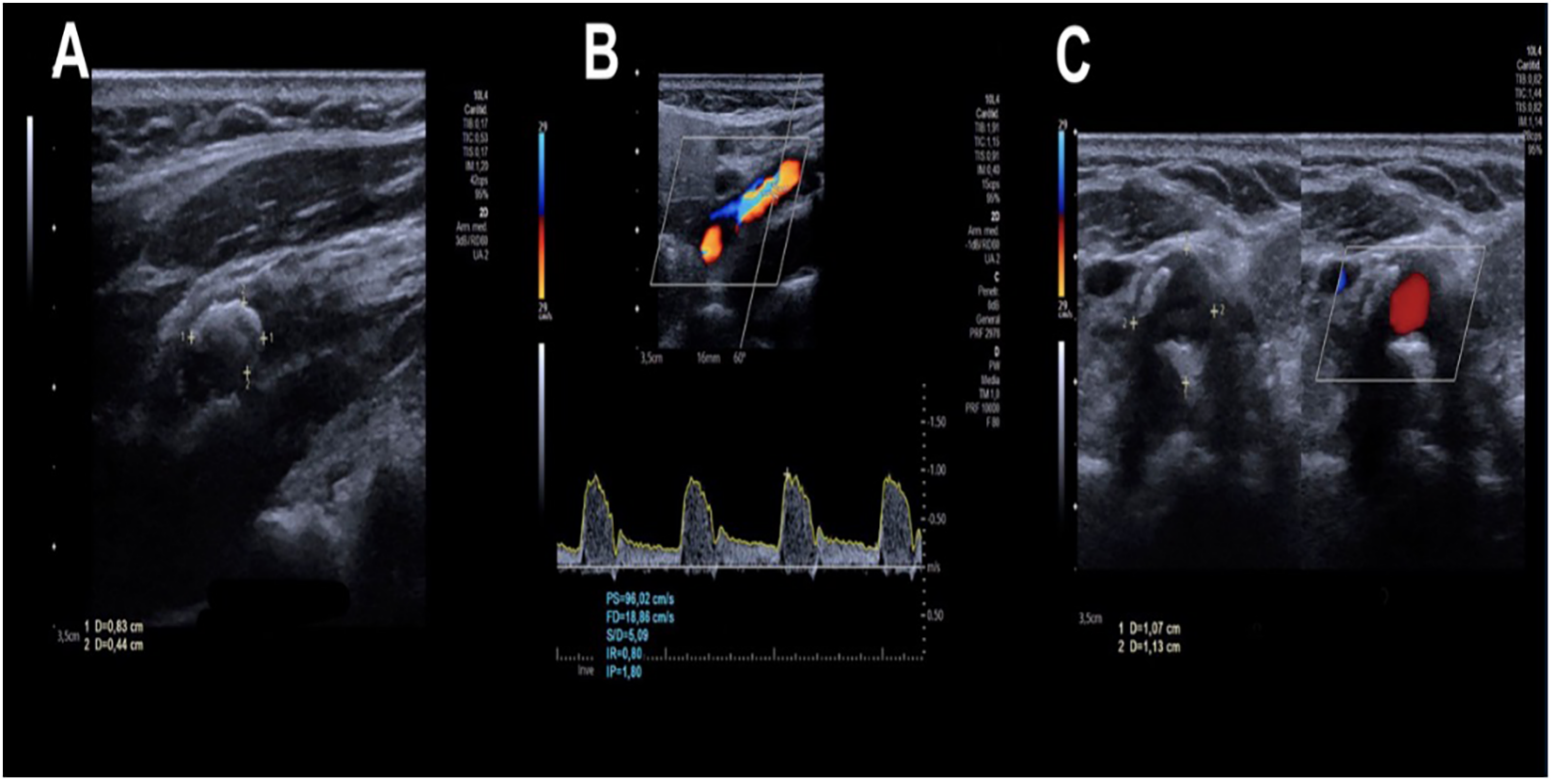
A carotid duplex ultrasound study of the right carotid artery of an 82-year-old patient was performed using real-time equipment with a multifrequency linear transducer in grayscale, color, and spectral Doppler. (A) At the level of the bulb, there is a plaque adjacent to the anterior and posterior wall, calcified, with extension toward the proximal segment of the internal carotid artery, measuring 9 mm and causing a significant decrease in vascular diameter. (B) Parameters measured at the level of stenosis: peak systolic (PS), diastolic flow (DF), systole/diastole ratio (S/D), and resistance indices (RIs). (C) Adjacent plaque to the posterior wall, calcified, measuring 10 mm and causing a significant decrease in vascular diameter. Image courtesy of Hospital Ángeles Universidad, Surgery Department. Used with permission.
